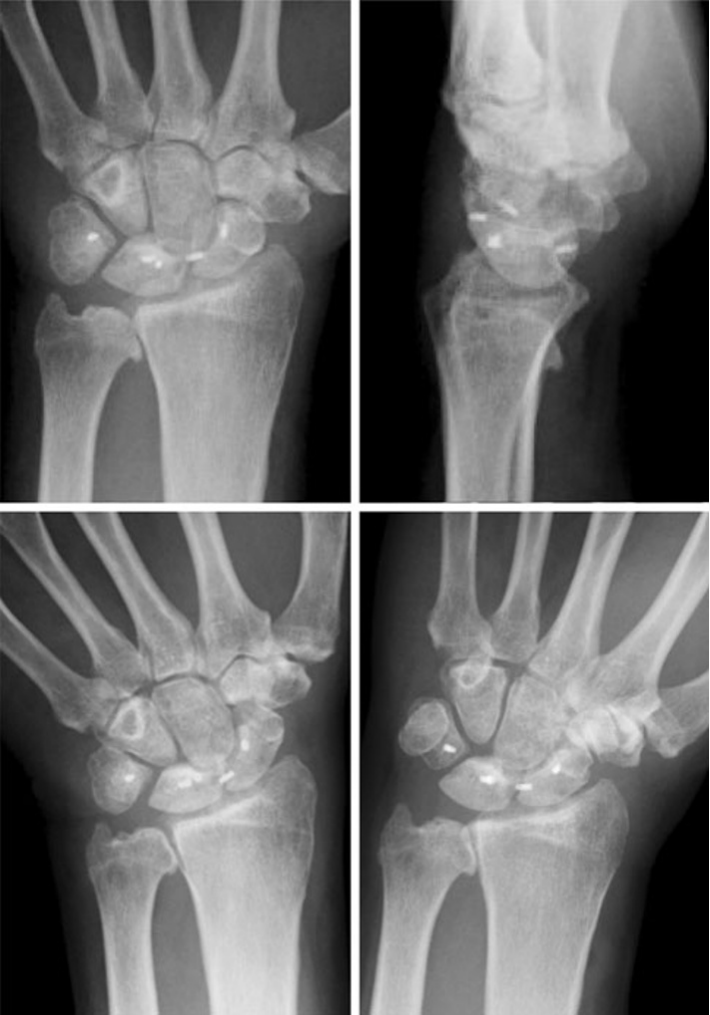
Radiographs at the 1-year follow-up. The scapholunate angle was 67° and the radiolunate angle 0°. Dorsal intercalated segment instability (DISI) deformity was not observed, although there was flexion of the scaphoid and a break in arc II of Gilula’s line at neutral and ulnar deviation
